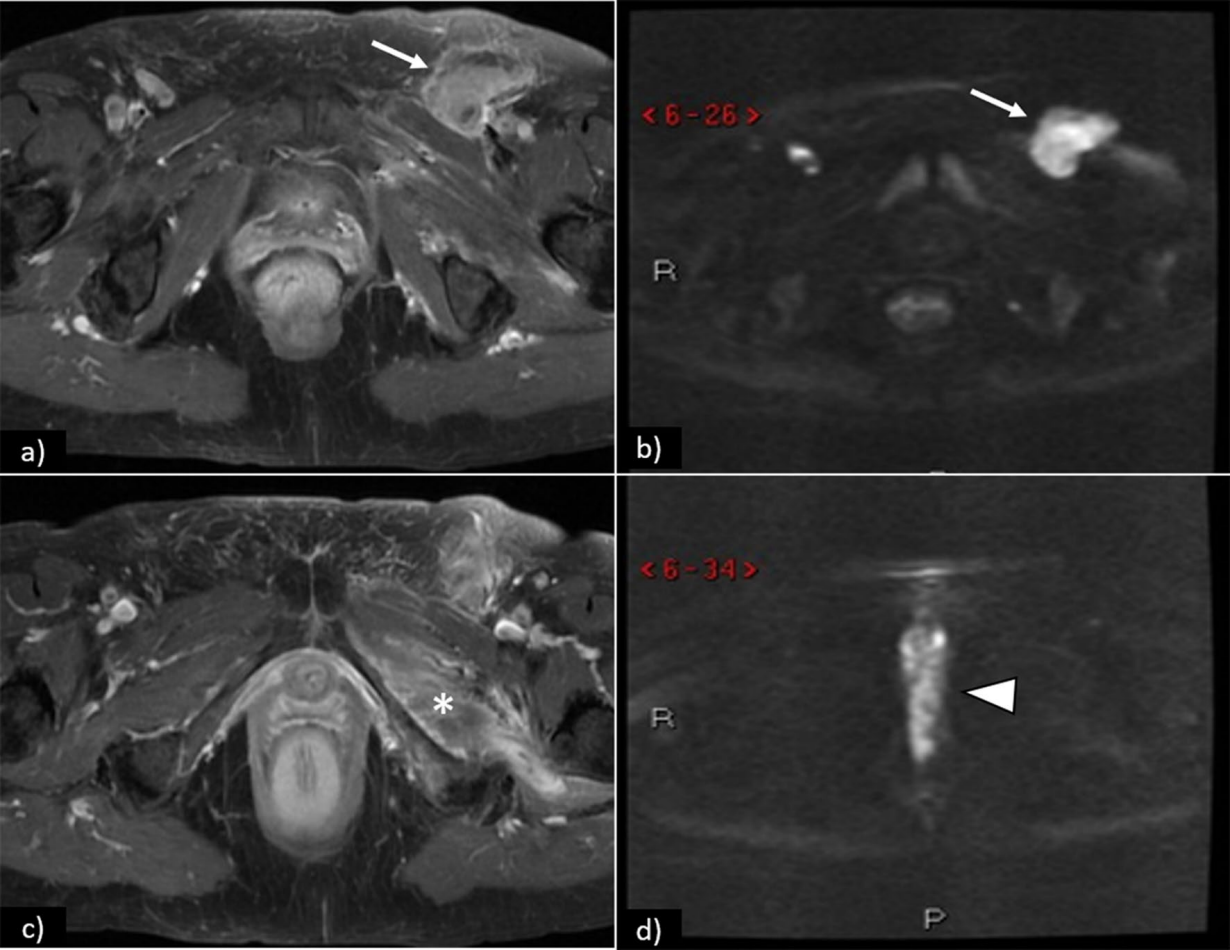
Axial contrast-enhanced T1WI a shows left inguinal lymphadenopathy (arrow) with restricted diffusion (arrow) on DWI (b). At different levels, axial contrast-enhanced T1WI (c) depicts left external obturator muscle involvement (*), and DWI (d) shows restricted diffusion of the primary vulvar tumour (arrowhead)
